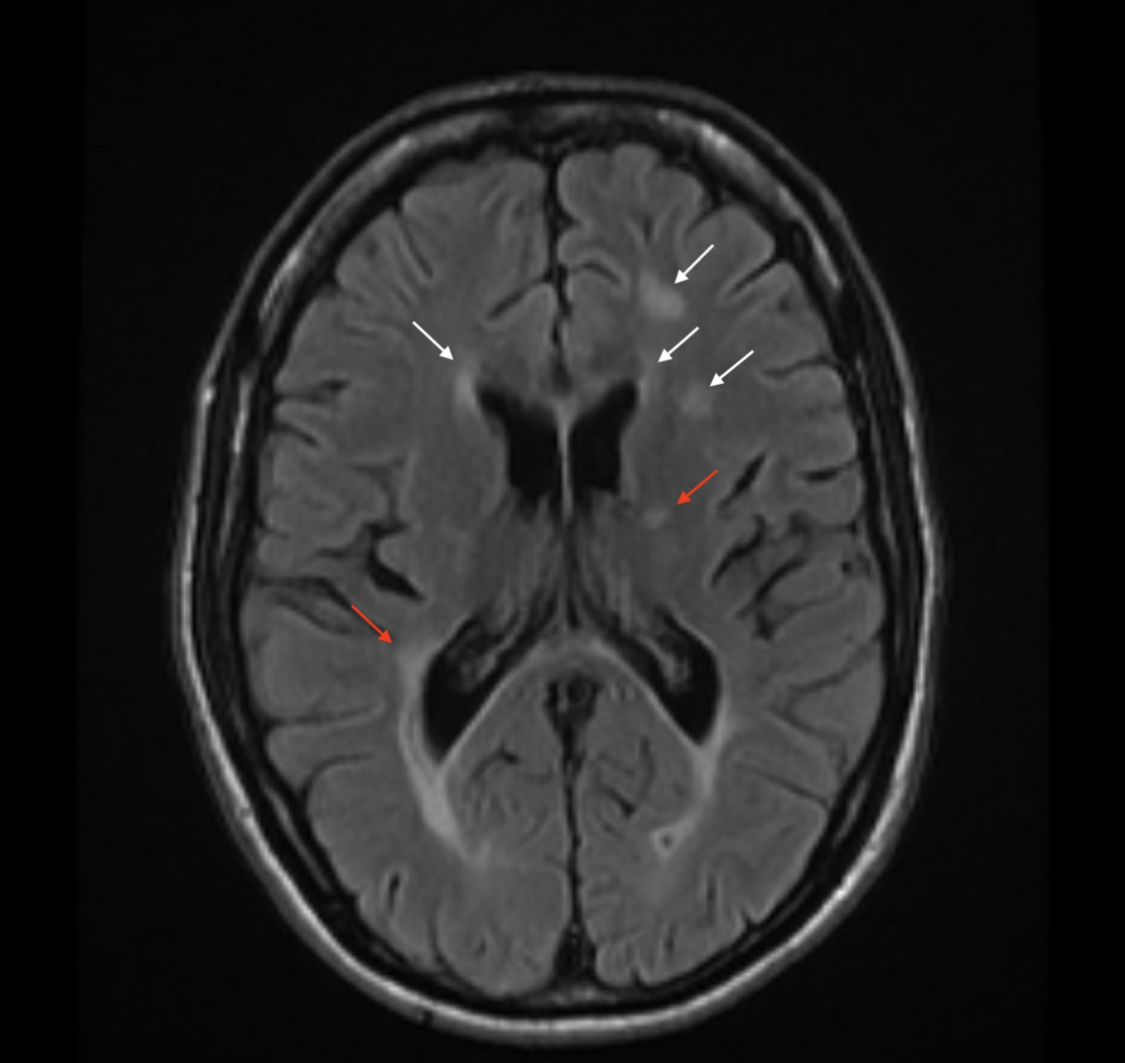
Axial T2 flair magnetic resonance imaging (MRI) of the brain revealing periventricular demyelinating process (white arrows) and white matter inflammatory changes around the perimedullary veins, known as Dawson Fingers (red arrows) consistent with multiple sclerosis
